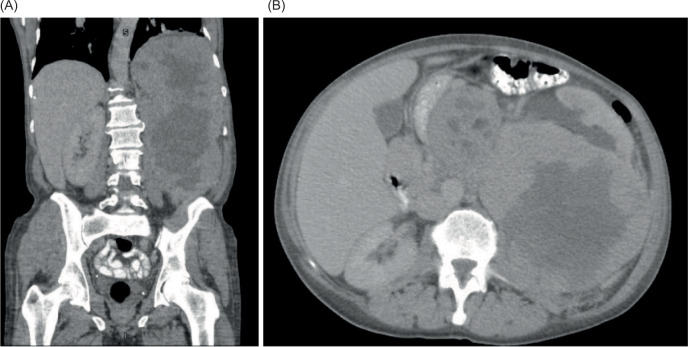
Abdominal CT images with contrast. CT images of the abdomen in coronal (A) and axial (B) planes with contrast enhancement demonstrating a large left retroperitoneal mass.
